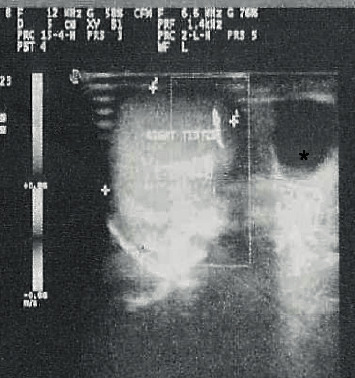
Ultrasound image of right testis with epididymal cyst^∗^ (on acute presentation).
